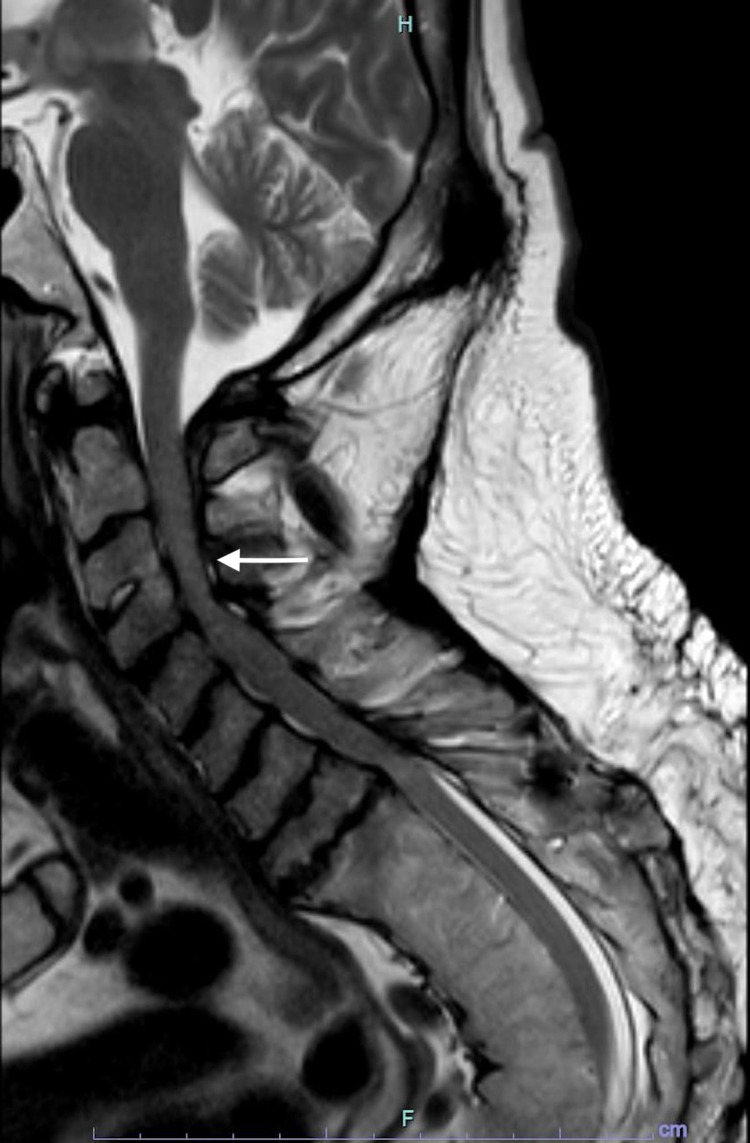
Preoperative MRI. Preoperative MRI indicating mild cord atrophy from C2 to C4, moderate stenosis at C2-C3 and C5-C7, and severe stenosis at C3-C5 (indicated by the arrow). In addition, disc herniation was noted at C4-C5 and C6-C7.
